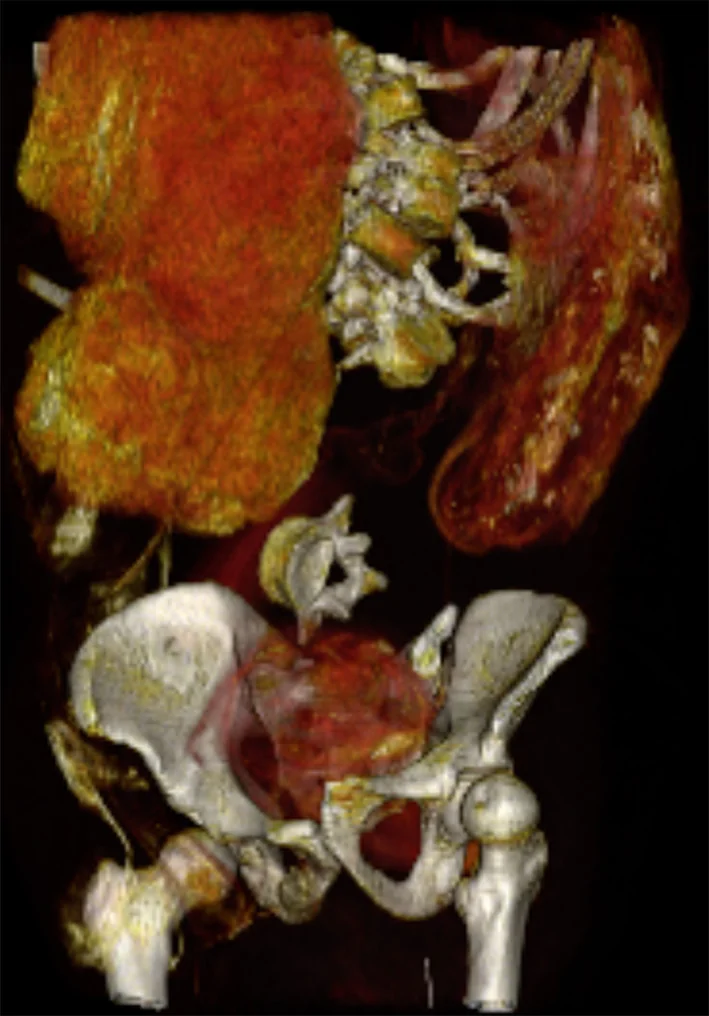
Coronal 3-dimensional CT image of lower torso of “Seqenenre-Taa-II” shows evisceration and embalming material within the abdomino-pelvic cavity. Note the disarticulated vertebrae, and disrupted symphysial articulation.
